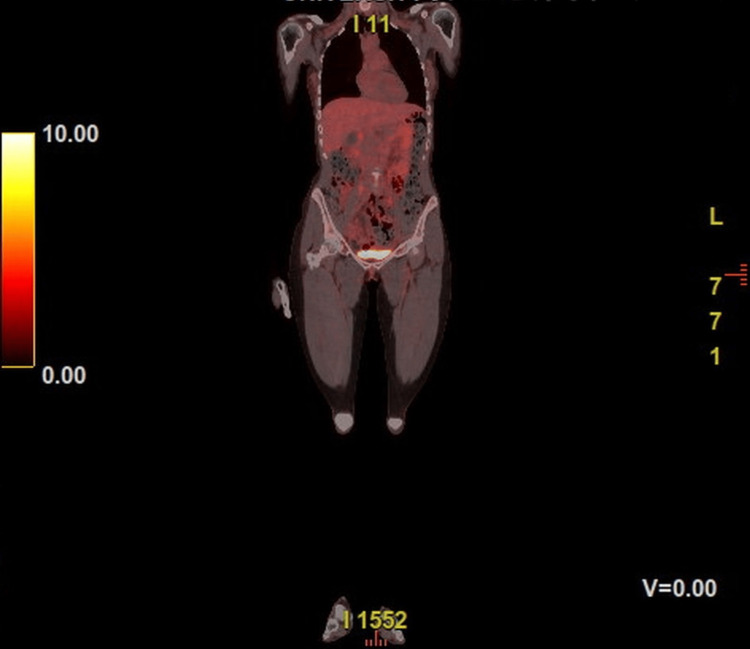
Coronal PET/CT scan performed postoperatively showing no evidence of distant metastatic disease.
